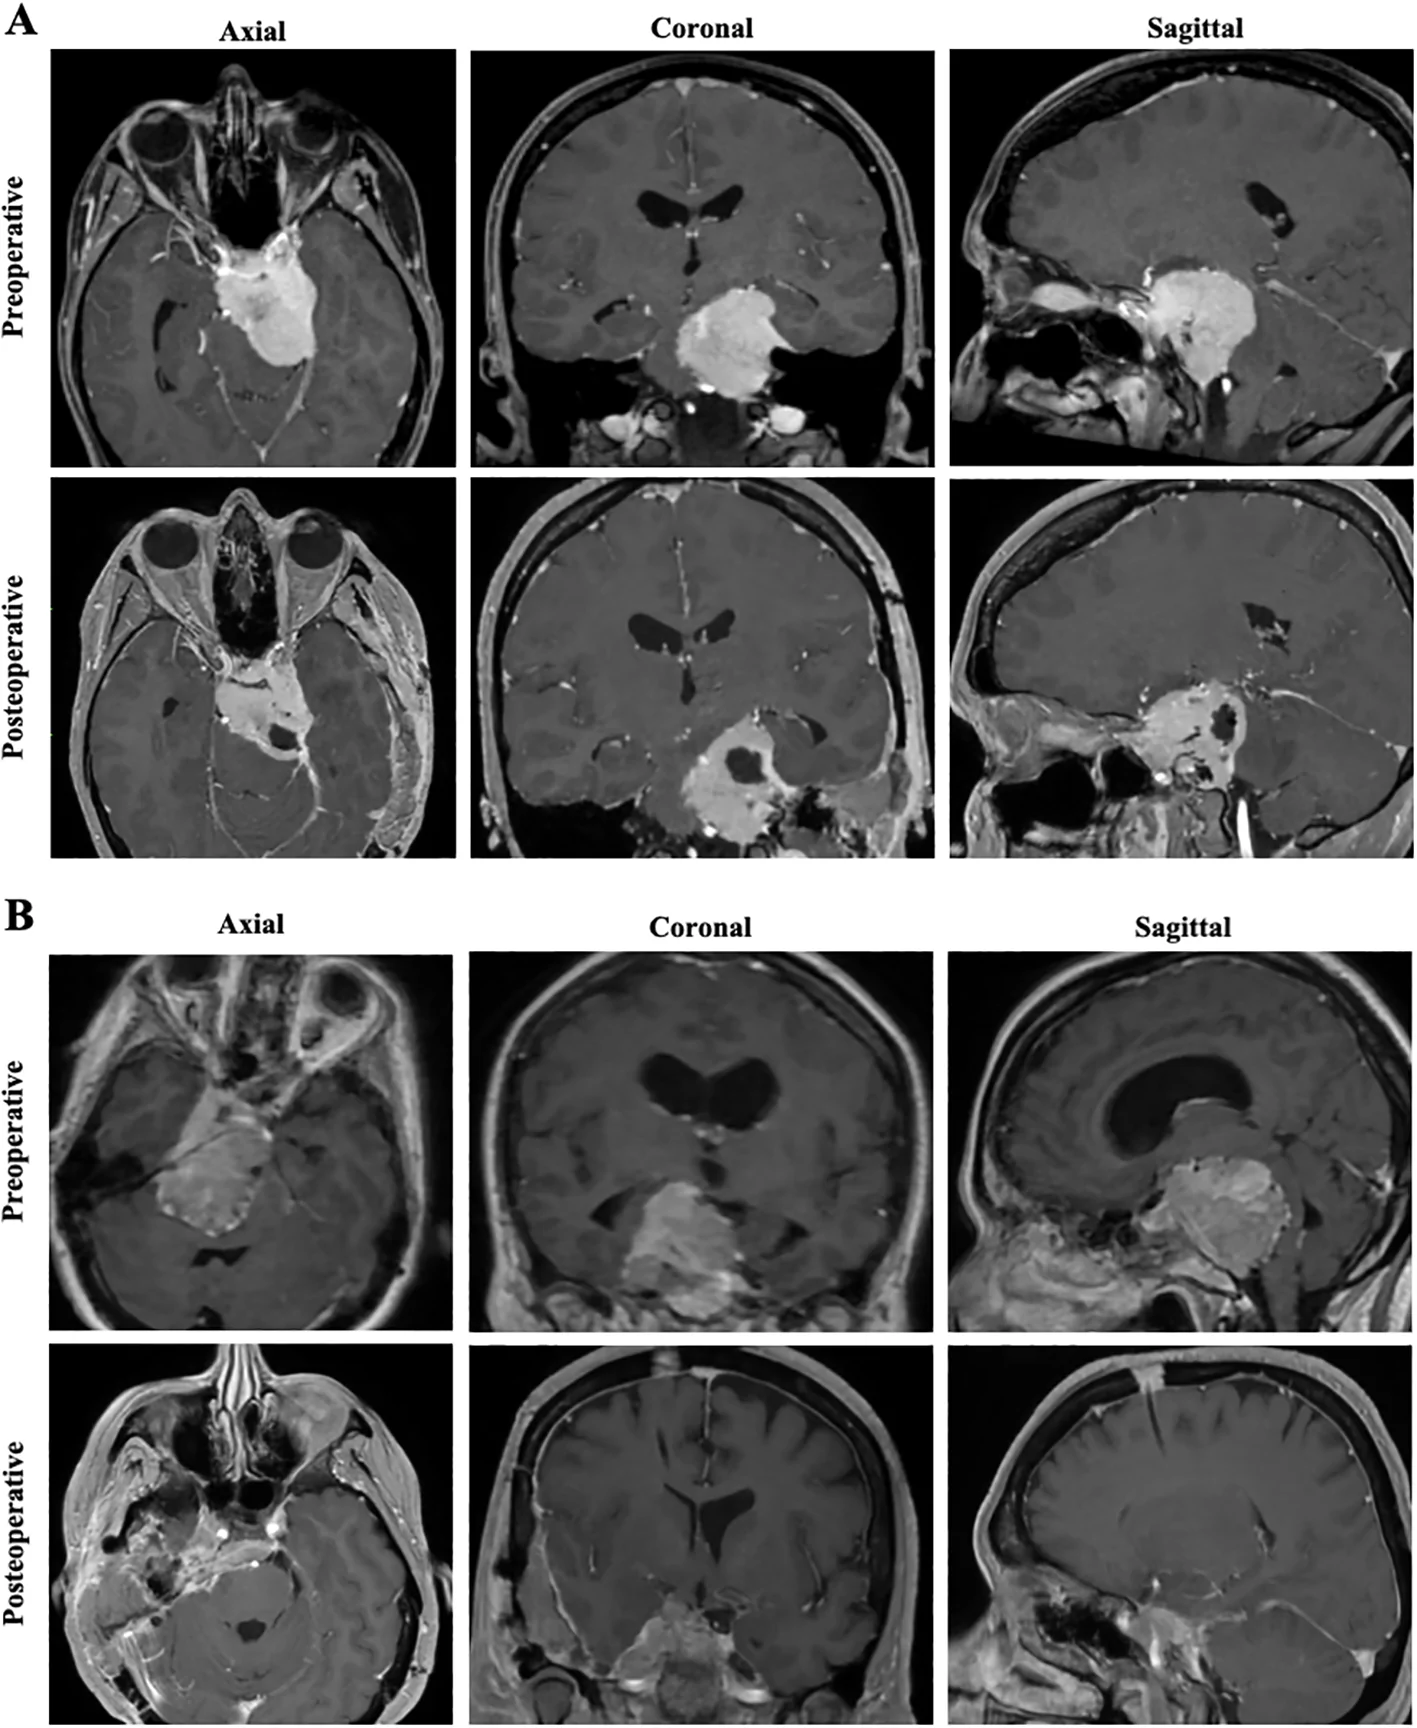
(A) Preoperative and postoperative MRI in the axial, coronal, and sagittal planes in patient 1: partial resection was performed because of the tumor firmness and its close adherence to the brainstem and neurovascular structures. (B) Preoperative and postoperative MRI in the axial, coronal, and sagittal planes in patient 2, showing a near-total resection with a small residual tumor (<10% of preoperative tumor) anterior to the pons and in the cavernous sinus.
